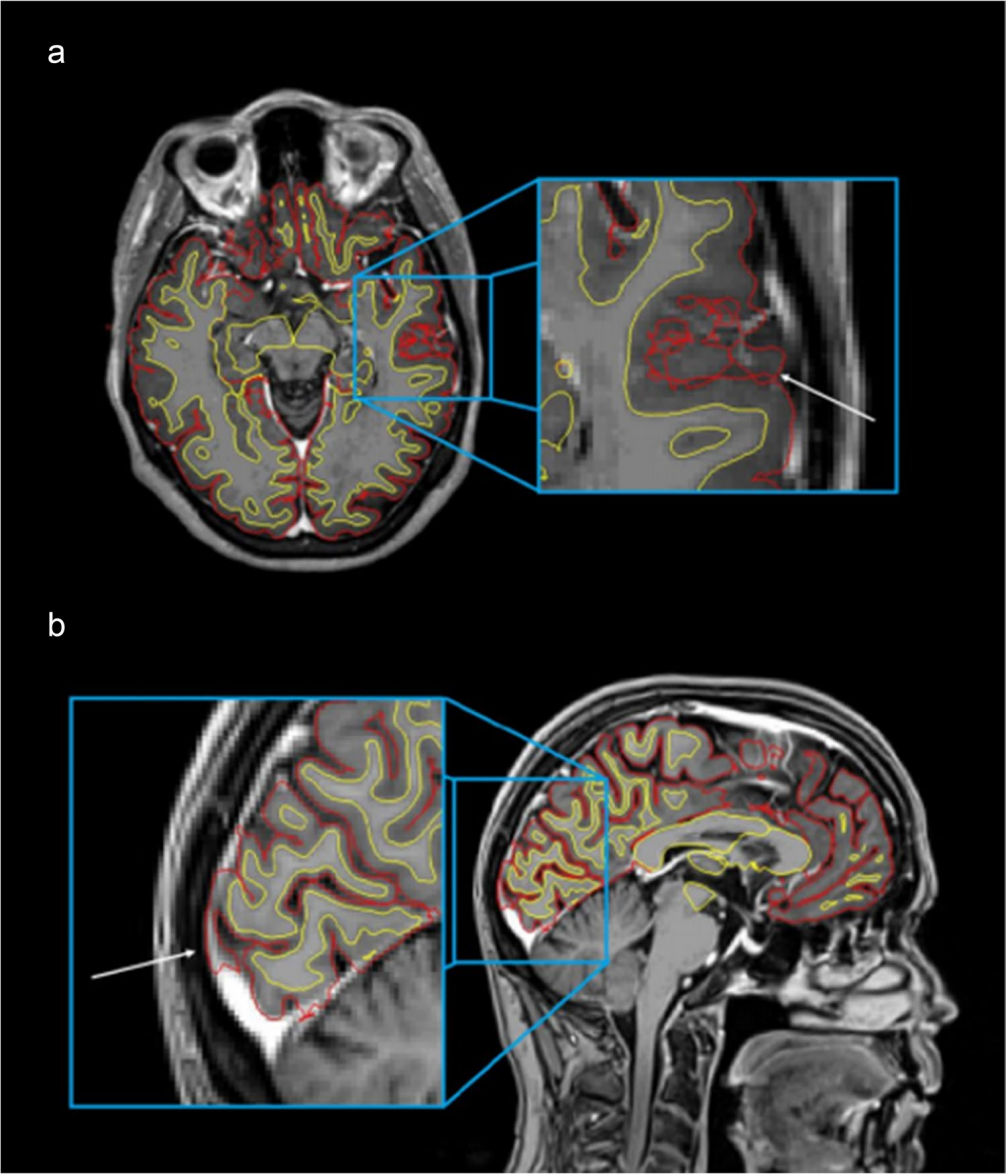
Post-contrast T1-weighted MRI, showing the border between WM and GM (white surface) (yellow), and the border between GM and CSF (pial surface) (red). a Axial slice showing a moderate pial surface “looping error” (white arrow). b Sagittal slice showing a typical skull stripping failure; a moderate error of the pial surface expanding into the dura and the sagittal sinus (white arrow)
